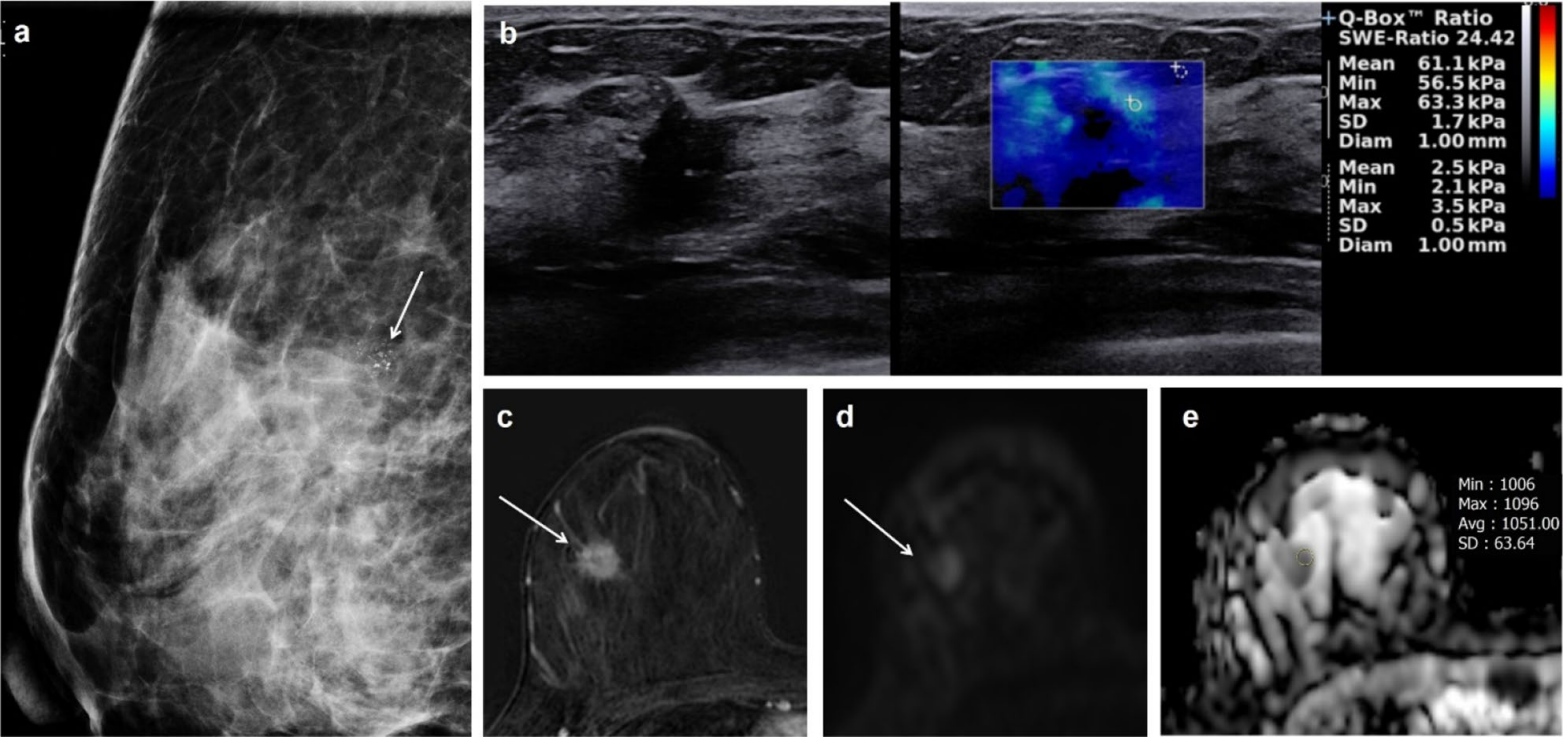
Imaging findings of a luminal A invasive breast cancer of no special type in the right breast of a 45-year-old woman. (a) Right magnification view shows suspicious microcalcifcations (arrow) in the upper outer quadrant. (b) B-mode ultrasound image (left) shows a 14-mm spiculated irregular hypoechoic mass containing calcifications. Shear-wave elastography (SWE, right) values are measured, with a mean elasticity of 61.1 kPa and a maximum elasticity of 63.3 kPa. (c) Axial contrast-enhanced T1-weighted subtraction magnetic resonance imaging (MRI) shows an irregular mass with heterogenous enhancement (arrow). The mass shows early rapid and delayed plateau enhancement. (d) Axial diffusion-weighted MRI (b value, 1000 s/mm^2^) shows a mass with high signal intensity (arrow). (e) On the reconstructed apparent diffusion coefficient (ADC) map, the ADC value of the mass is 1.048 × 10^−3^ cm^2^/s. US-guided core-needle biopsy revealed invasive breast cancer of no special type that was estrogen receptor positive, progesterone receptor positive, and human epidermal growth factor receptor 2 positive.
